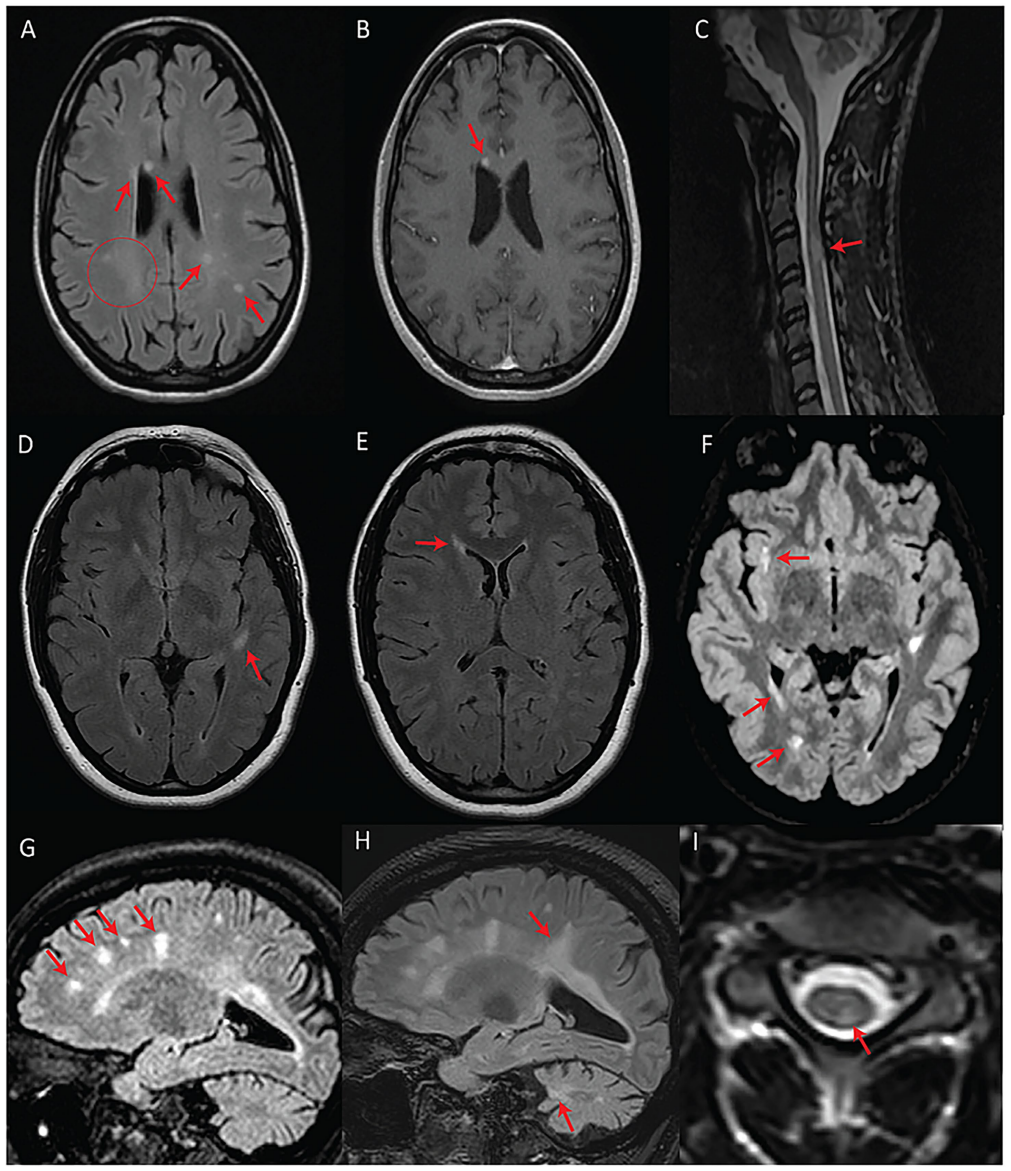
Patients with fulfilled or partially fulfilled McDonald criteria. (A–C) Represent subject #2 (criteria fulfilled). (A) Axial T2-FLAIR image showing multifocal periventricular, callosal, and subcortical white matter lesions (arrows) with accompanying diffuse abnormal peritrigonal white matter signal (circle). (B) Post-gadolinium axial T1 image showing an enhancing callosal lesion. (C) Sagittal T2 image of the cervical and thoracic spinal cord showing a demyelinating lesion at the level of C3. (D–F) Represent subject #1 (criteria partially fulfilled). (D) Axial T2-FLAIR showing an oval-shaped lesion in the left temporal subcortical white matter. (E) Axial T2-FLAIR showing a periventricular lesion around the frontal horn of the right lateral ventricle. (F) Axial T2-FLAIR, performed 7 years after the first exam (D,E), showing new focal white matter lesions in the right insula, right periventricular white matter, and along the posterior horn of the right lateral ventricle. (G–I) Represent subject #3 (criteria fulfilled). (G) Sagittal T2-FLAIR showing multifocal white matter lesions perpendicular to the ventricular walls. (H) Sagittal T2-FLAIR, performed 4 years after initial MRI (G), demonstrating progression of the lesion burden in both the supra and infratentorial regions despite treatment with teriflunomide as of the first year in the 4-year interval. (I) Axial T2 spinal cord MRI showing a white matter lesion located in the left posterior cord at the C3 level.
